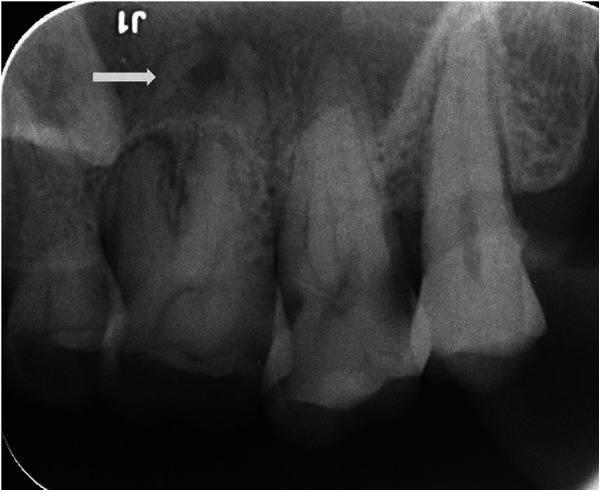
Periapical radiograph of the left maxillary first molar presented with a periapical radiolucency involving the apex at the palatal root and associated cemental tear.
